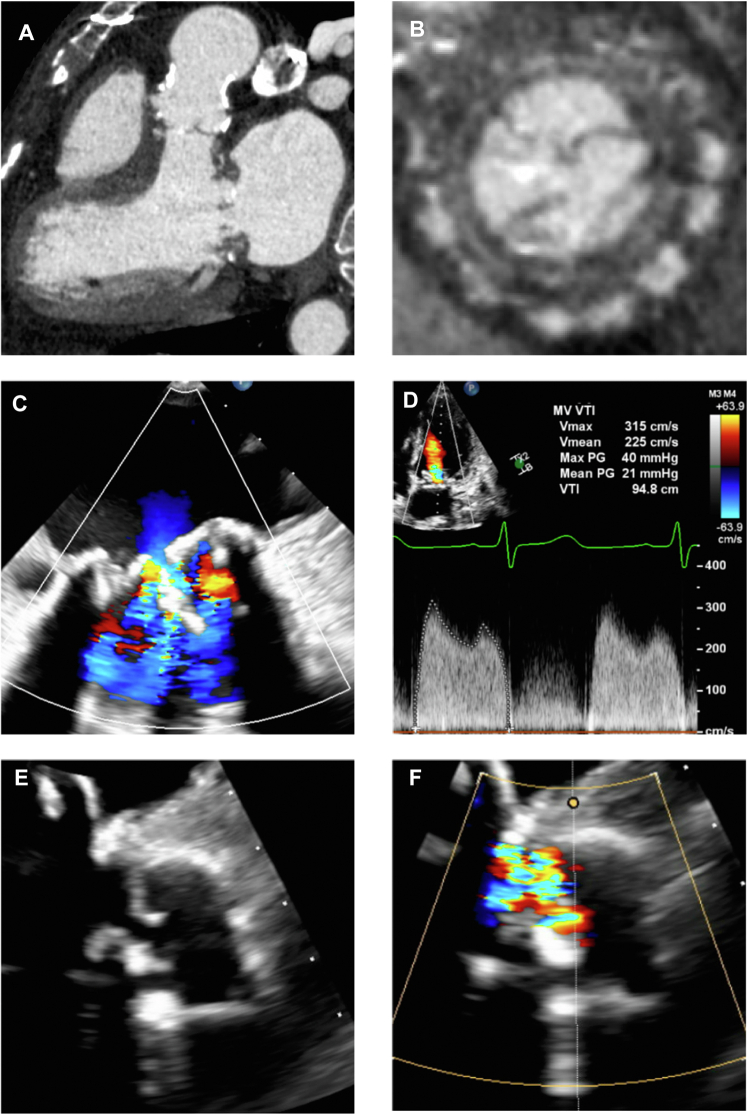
Multimodality Imaging Evaluation Cardiac computed tomography showed extensive calcification of the Freestyle aortic root and aortic valve bioprosthesis (A) and bioprosthetic mitral valve (B), without evidence of valve thrombosis. Transesophageal echocardiography showed bioprosthetic mitral valve leaflet thickening with significantly decreased excursion (C) and mild to moderate regurgitation. Before surgery, transthoracic echocardiography showed severe bioprosthetic mitral valve stenosis with diastolic mean gradient of 21 mm Hg (D). Transesophageal echocardiography also revealed severe bioprosthetic aortic valve regurgitation secondary to a flail anterior cusp (E, F).
